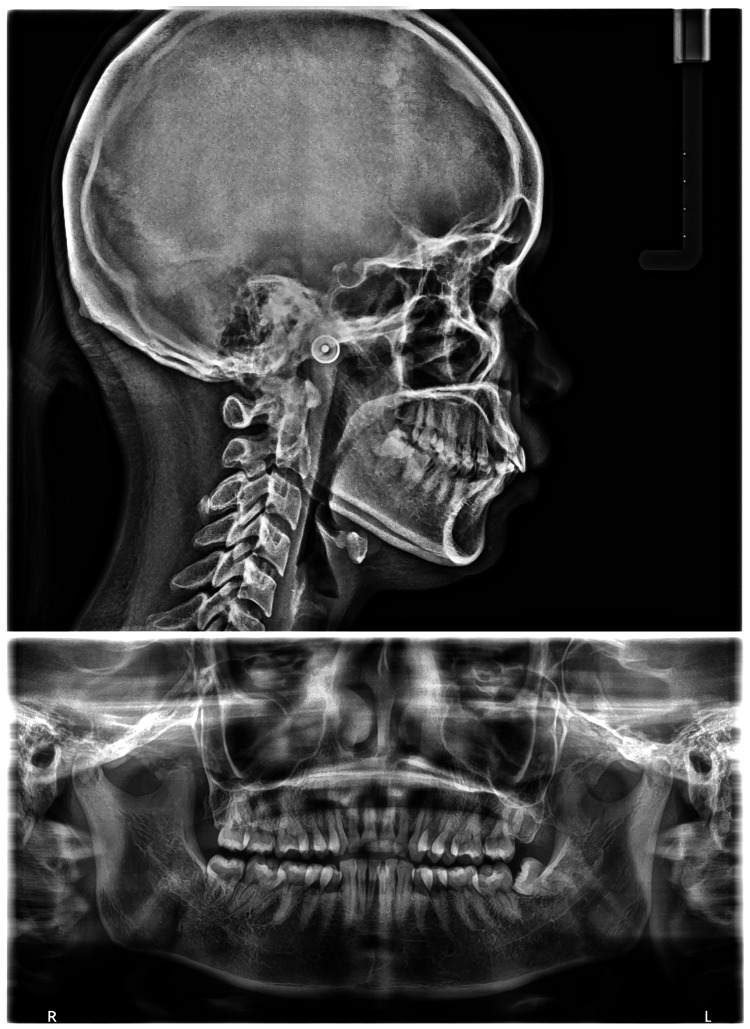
Representative Lateral Cephalogram and Panoramic Radiograph De-identified sample lateral cephalogram and panoramic radiograph demonstrating the imaging views used for cephalometric tracing and classification of mandibular third molar impaction. These images were taken in the department of Dentistry, Tata Main Hospital and contain no patient-identifiable information.
